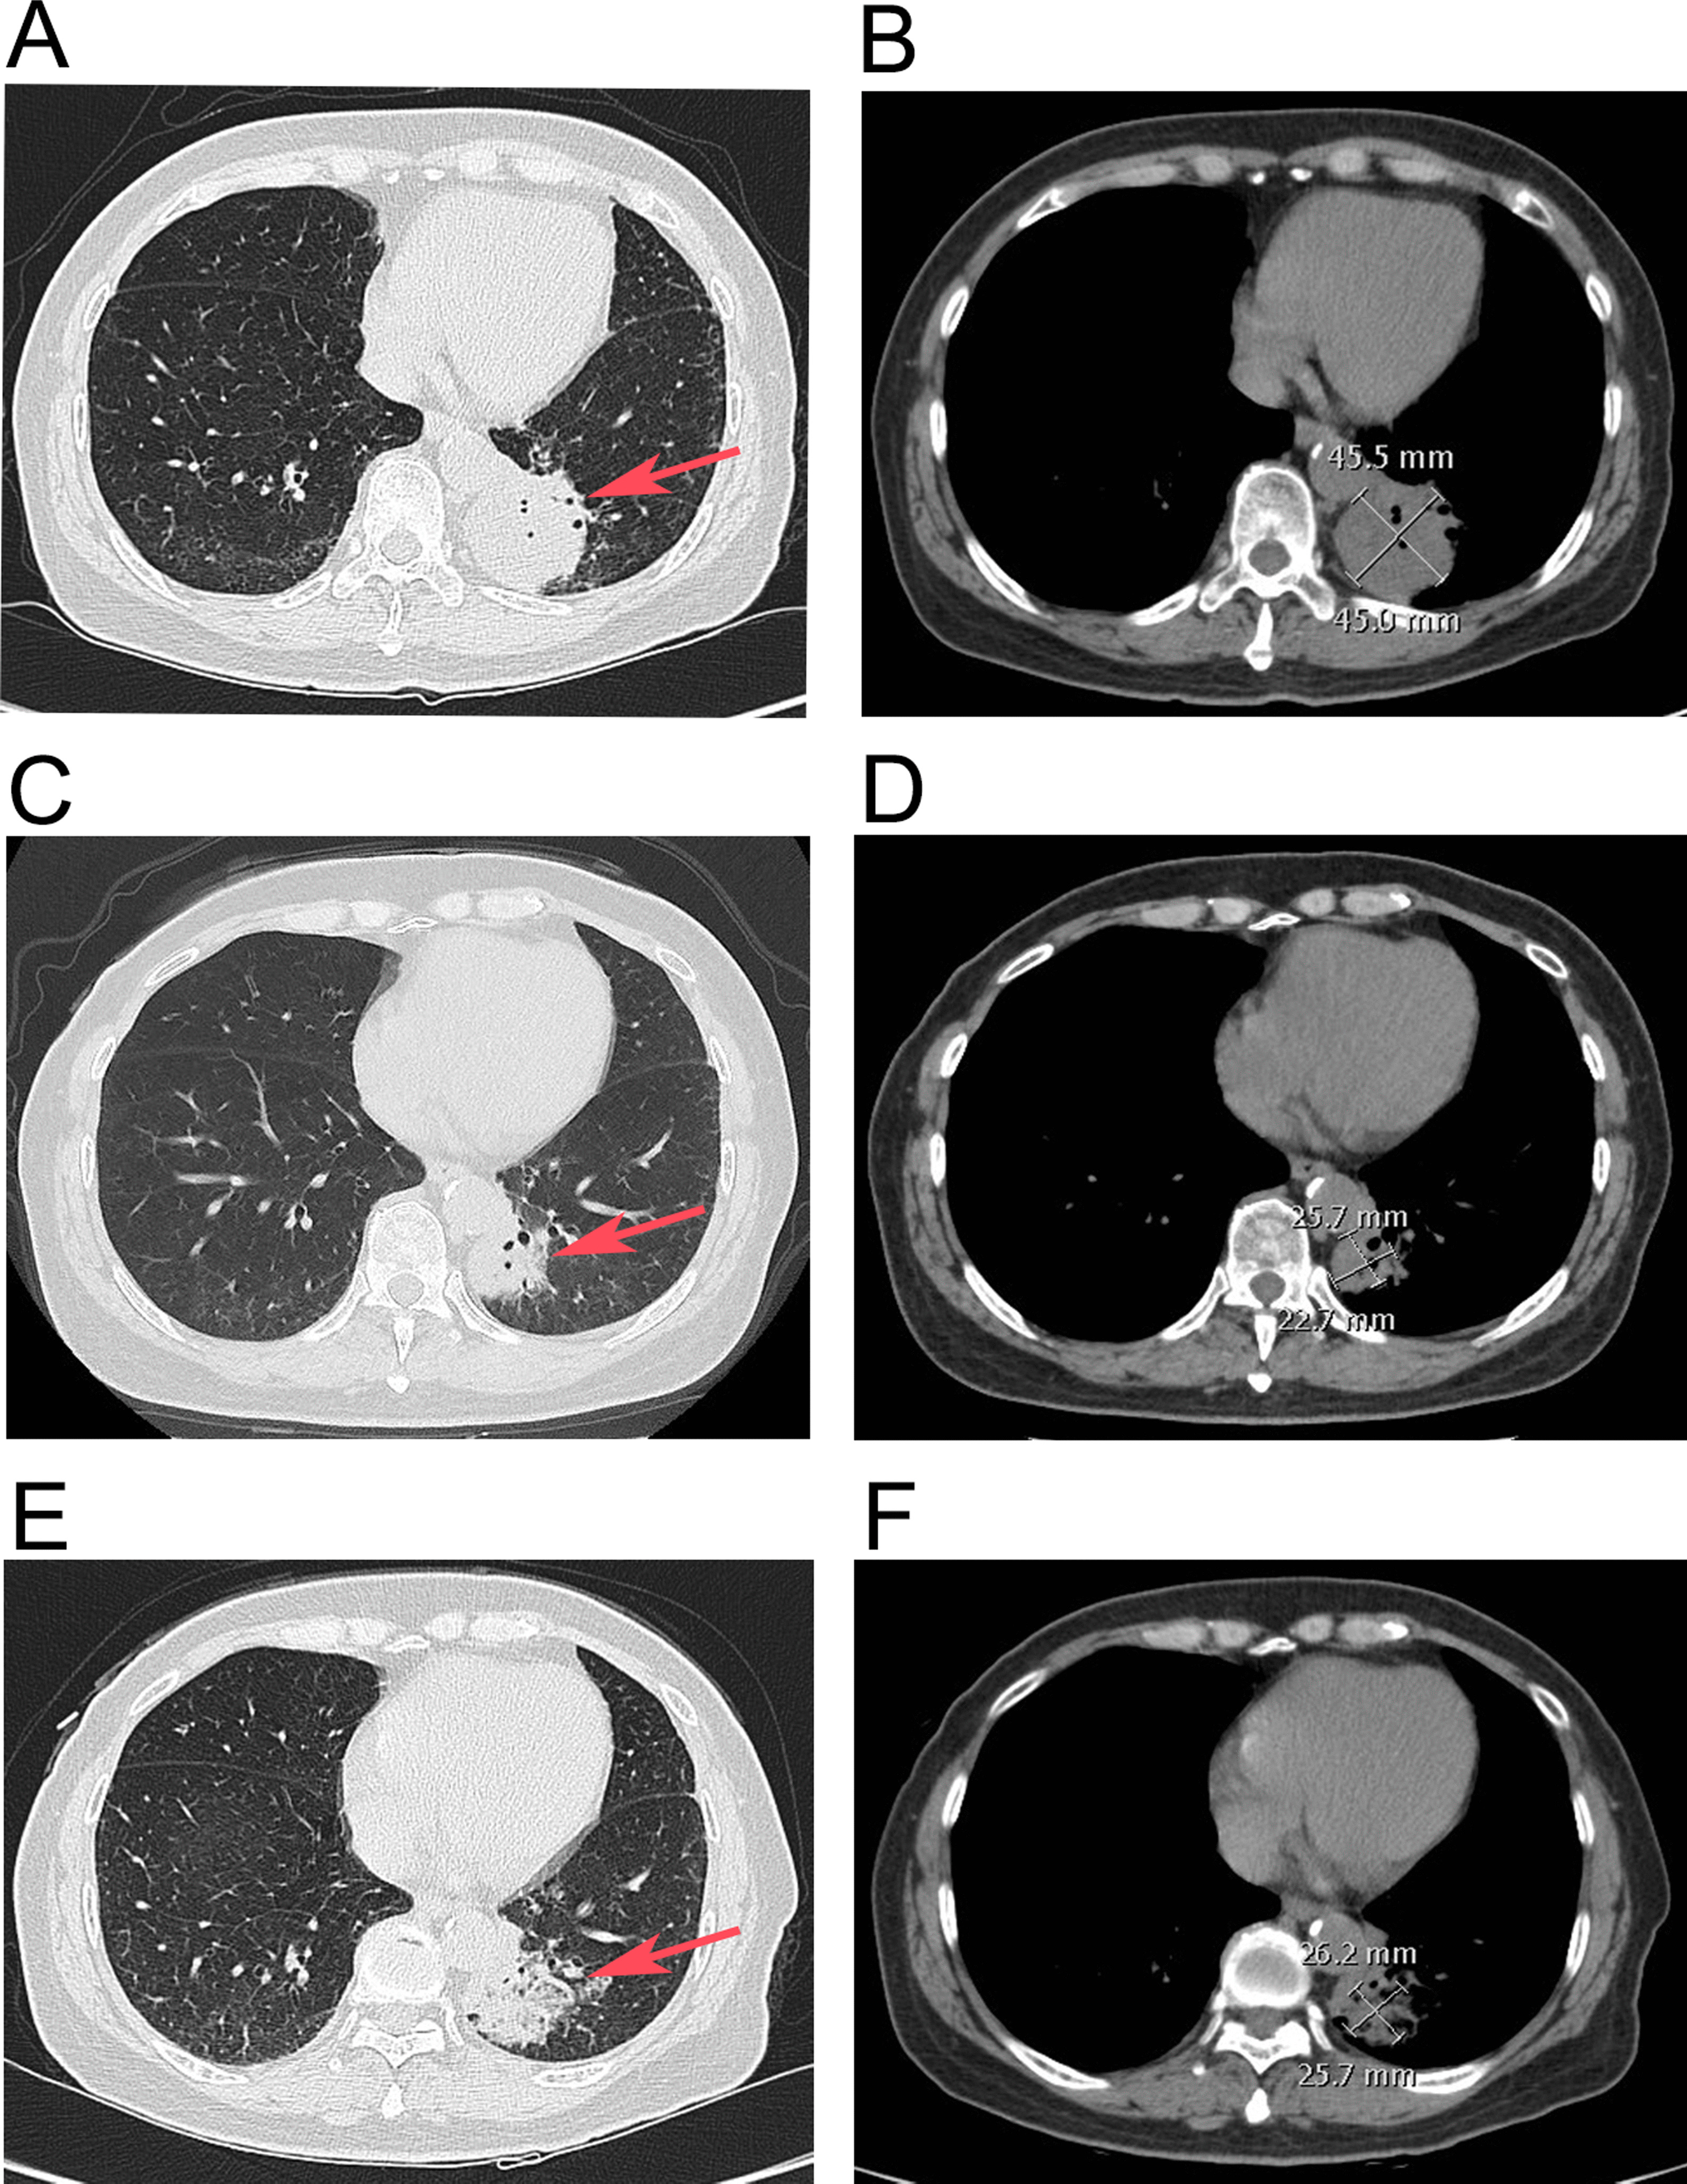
The change of chest computed tomography (CT) image. a, b The chest CT showing a mass (45.5 × 45.0 mm^2^) in the left lower lobe before treatment of penicillin. c, d The chest CT showing narrowed mass (25.7 × 22.7 mm^2^) in the left lower lobe after treatment of penicillin for 2 months. e, f The chest CT showing a mass (26.2 × 25.7 mm^2^) with expanded ground glass opacity in the left lower lobe after treatment of penicillin for 4 months. a, c, e pulmonary window, red arrow: mass; b, d, f: mediastinal window)
